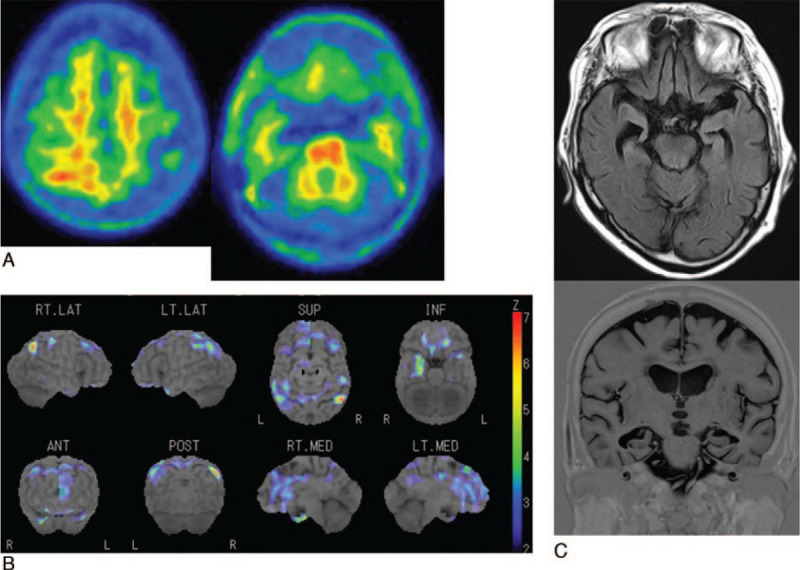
A 81-year-old woman with vascular dementia (Case 23). a. ^11^C-PiB PET shows negative amyloid burden with, a mean cortical standardized uptake value ratio (SUVR) of 1.40. b. ^123^I-IMP SPECT and color-coding representing the statistical significance of decrease in regional cerebral blood flow as compared to normal shows hypoperfusion in both the parietotemporal regions, posterior cingulate gyrus and precuneus. c. Axial and coronal MRI shows severe atrophy of both hippocampus, with a Z-score of 3.48.
